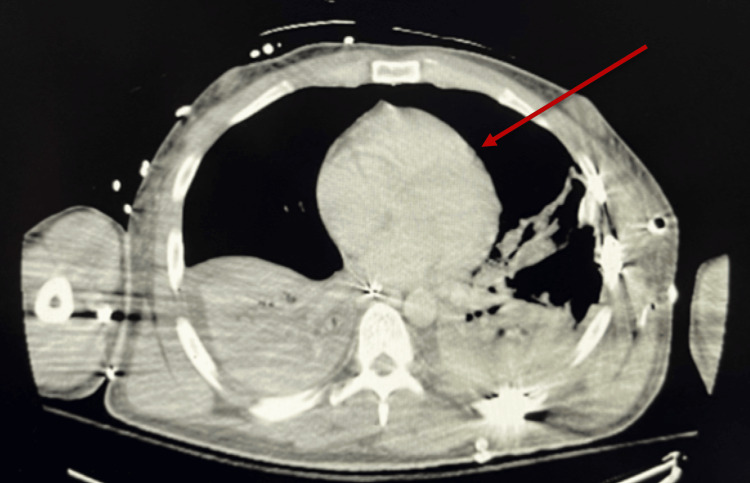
Chest CT on admission showing no evidence of pericardial effusion The red arrow shows normal pericardium and lack of effusion.
